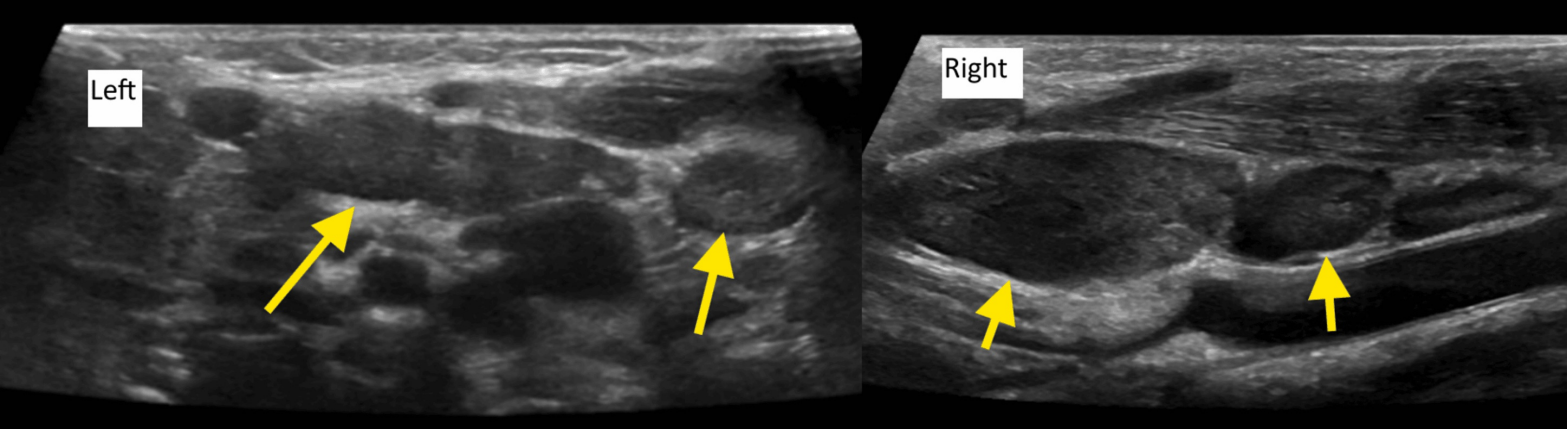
Neck ultrasonography showing left-sided (Left) and right-sided (Right) lymphadenopathy (arrows)
